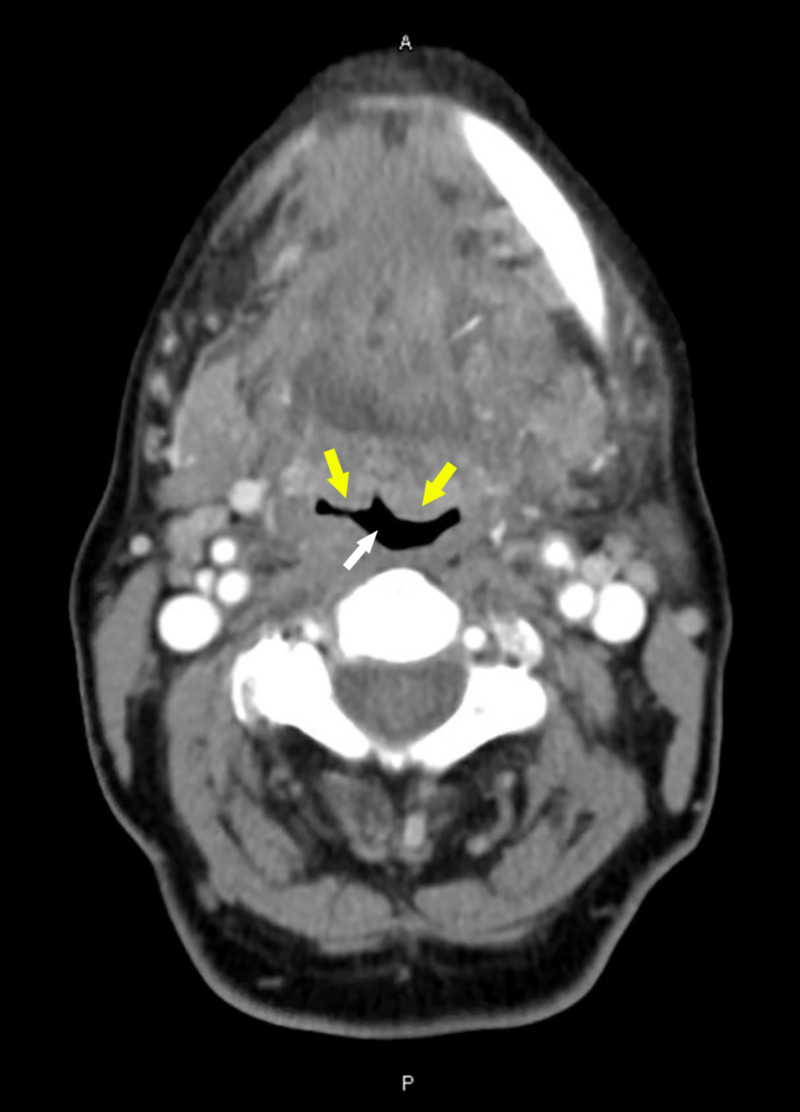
CT of the neck soft tissue revealing LTH (yellow arrows), almost collapsing the airway (white arrow). Only a small crescent-shaped region of the airway remains patent. LTH, lingual tonsillar hypertrophy
